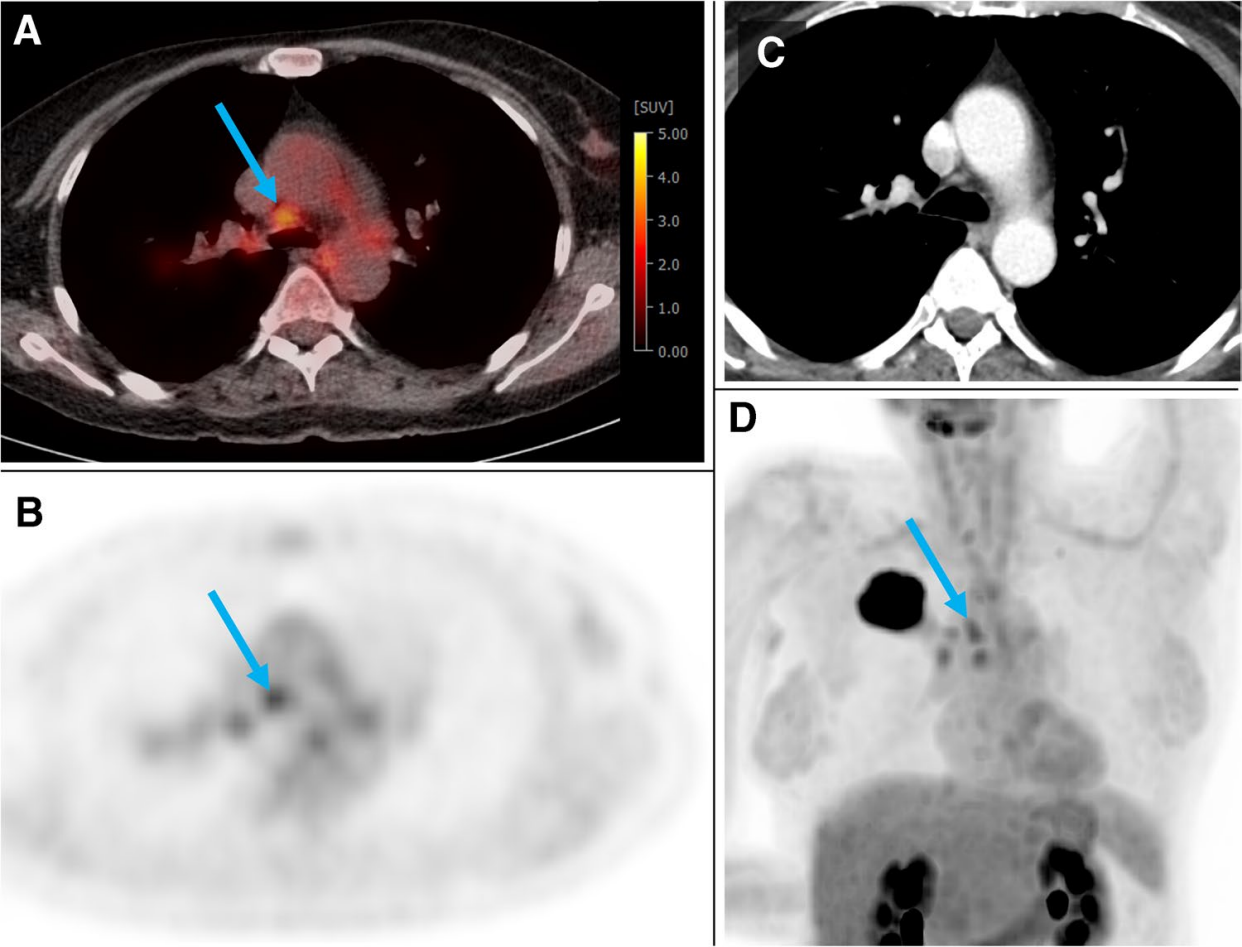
Case example #1. Fifty-four-year-old woman from the training + test cohort with a G2 adenocarcinoma of the right upper lobe (44 mm). Several hilar and mediastinal lymph nodes (LN) showed higher [^18^F]FDG uptake than the normal liver (= PET score of 3).The blue arrow depicts an N2 paratracheal LN with 7 mm short axis and SUVmax of 3.5. Based on visual assessment, N2 disease would be suspected in this case. According to the GBM model, probability of N2/3 is only 0.17 (= negative). The patient was confirmed to be N0 by ultrasound-guided transbronchial needle biopsy of the ipsilateral hilar, paratracheal and subcarinal LNs
